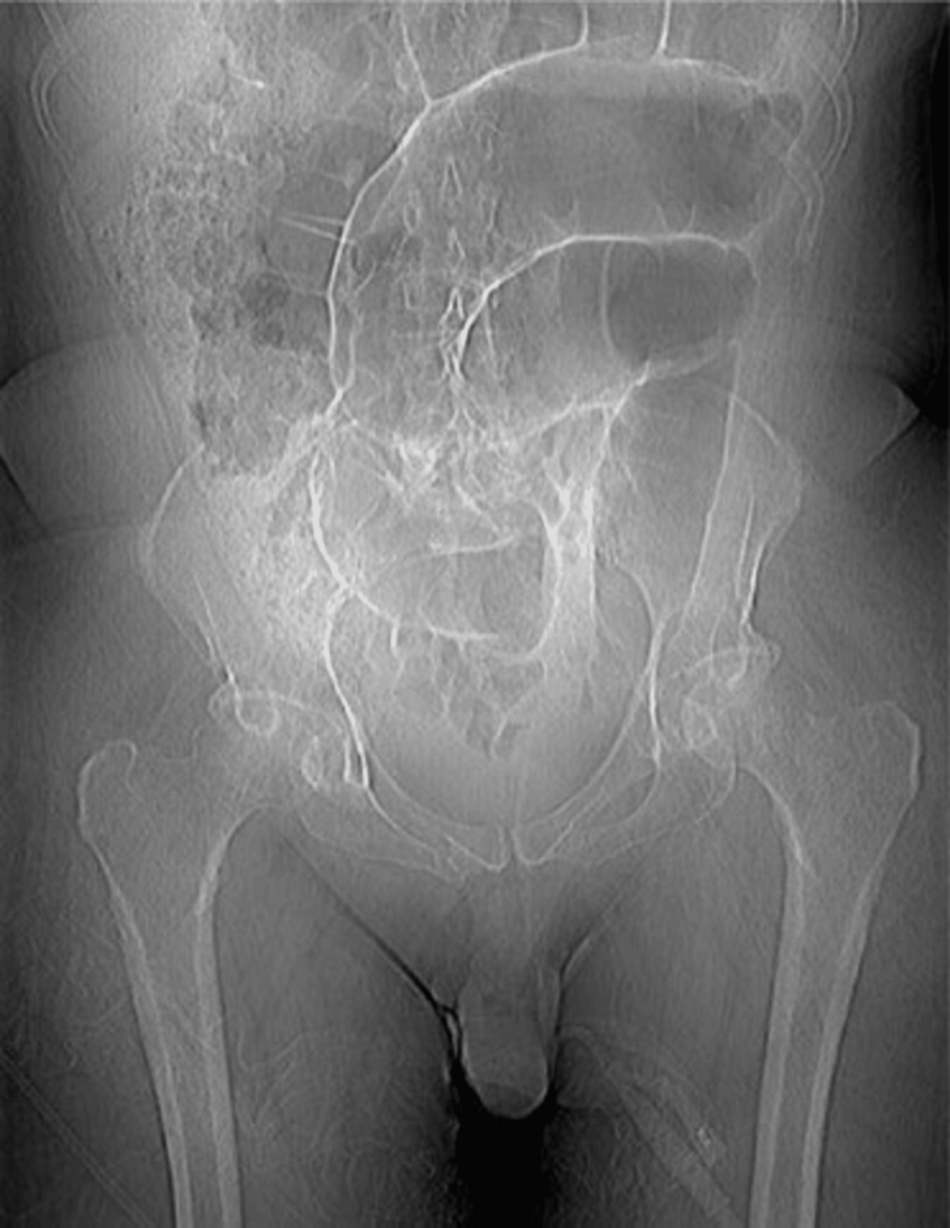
CT of the abdomen with contrast showing bowel distension and not revealing any specific pathology
